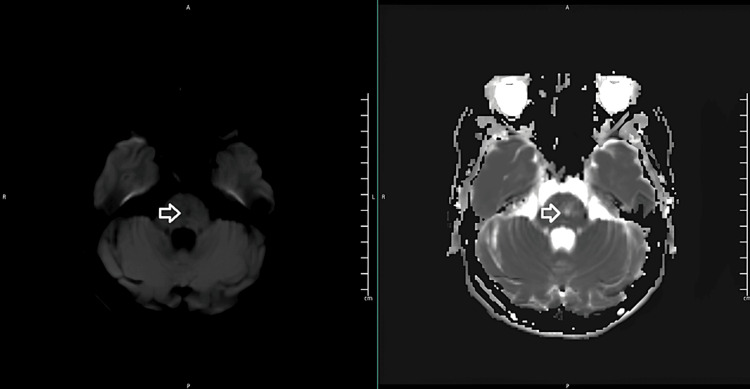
An MRI head DWI (left) and ADC (right) images showing a left paramedian pons lacunar infarct. DWI - Diffusion-weighted imaging, ADC - apparent diffusion coefficient
